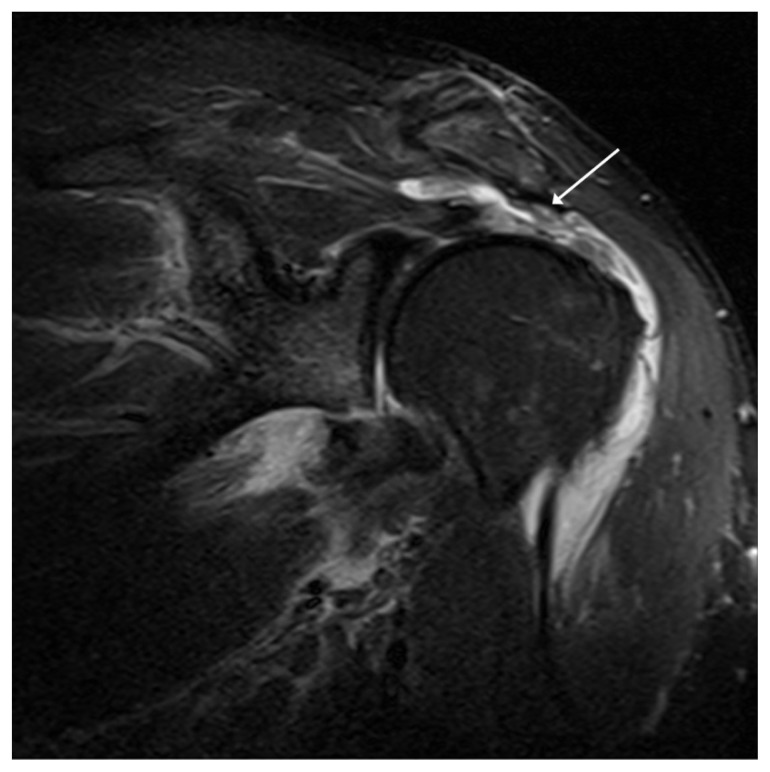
Magnetic resonance imaging (MRI) showed large retracted supraspinatus tendon avulsion following traumatic dislocation.
